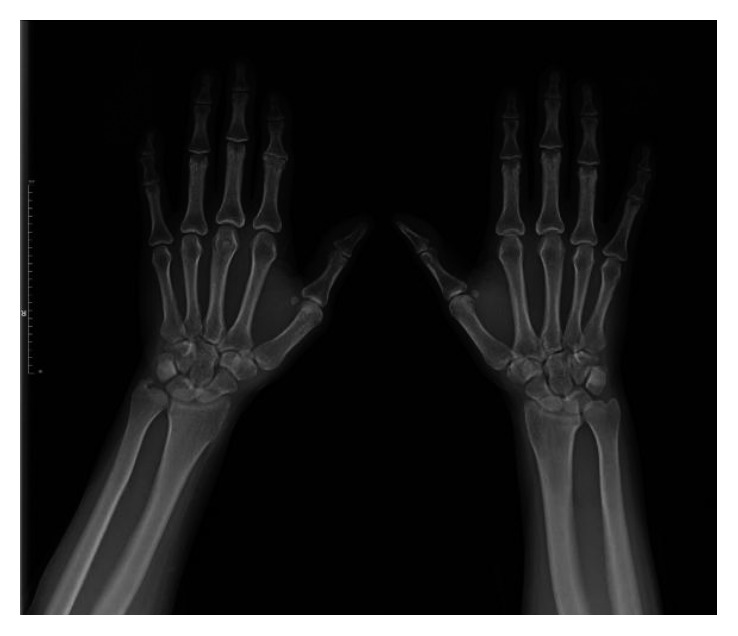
X-ray findings of the hands (patient 2).
